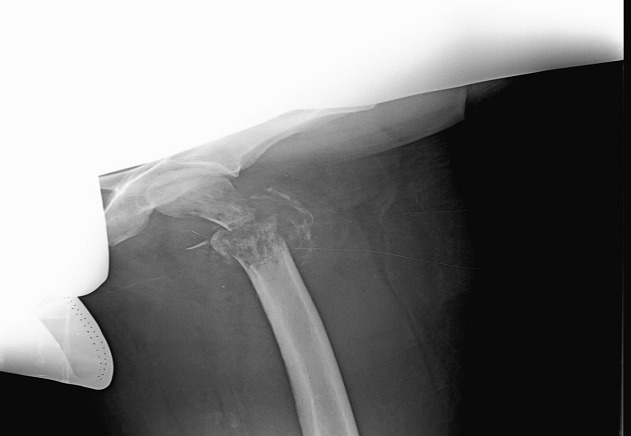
Standard Radiography of the left thigh from the front indicates an osteolytic image at the level of the metaphyseal region of the femur complicated by a displaced pathological pertrochanteric fracture
